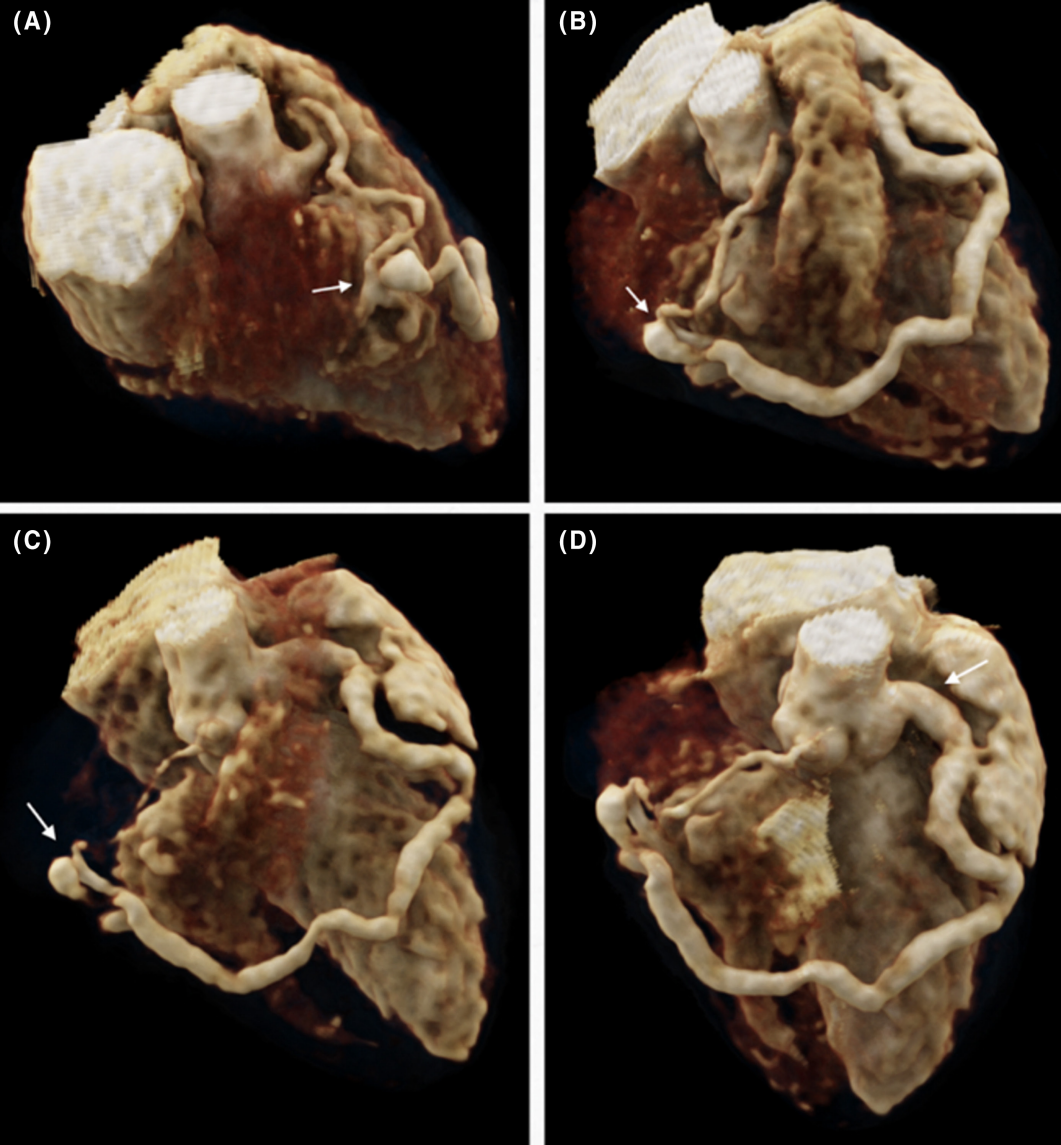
Coronary CT angiography volume render reveals the following observations: (A) right coronary artery (RCA) proximal tortuosity and midsegment dilation, where it joins the left anterior descending artery (LAD) fistula prior to its drainage into the right ventricle (RV). (B, C) The “arched” trajectory of the LAD coronary fistula and its connection with the RCA is evident, with its entry point situated at the basal third of the RV free wall. (D) Dilation of the left main coronary artery and the LAD, abnormal path of the LAD up to its convergence with the RCA.
